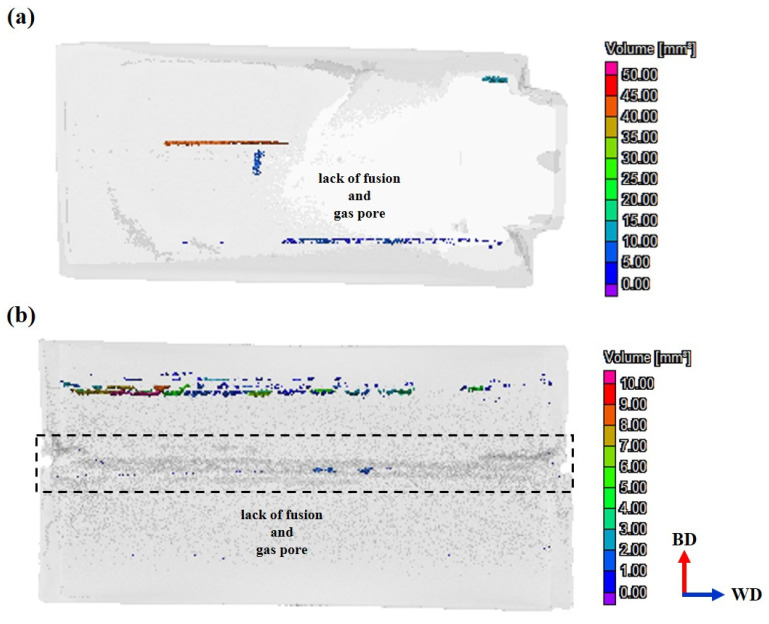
Image of 3D X-ray CT scan analysis: (a) sample A, (b) sample A-W.
